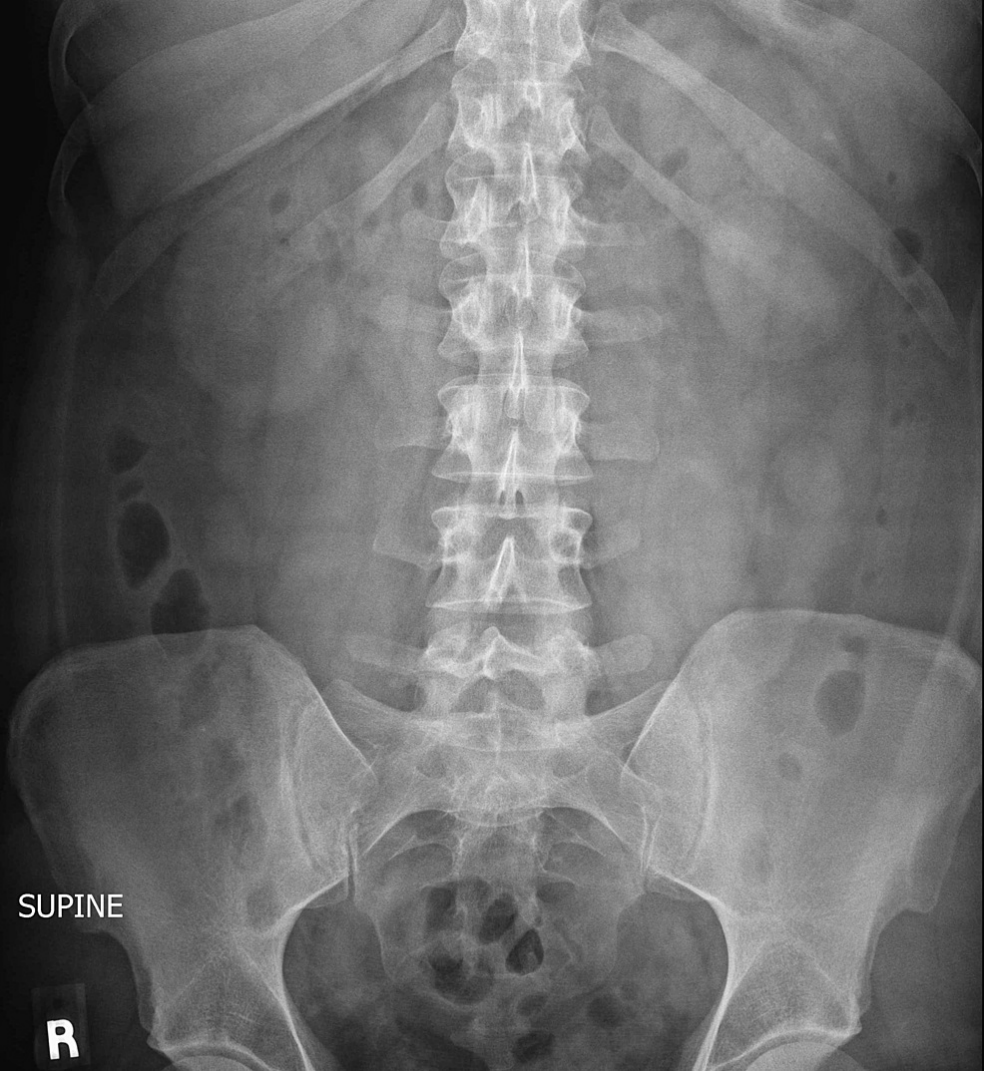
AP abdominal X-ray showing no bowel obstruction or other remarkable findings.
